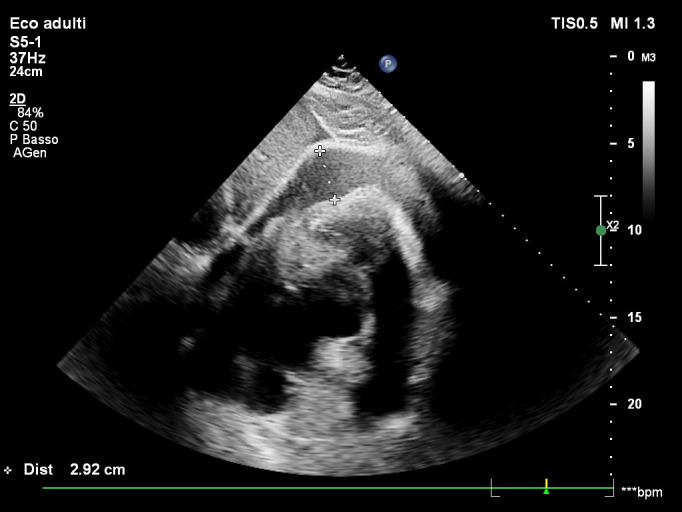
Transthoracic echocardiography (subcostal view) showing a large circumferential pericardial effusion. Subcostal TTE image demonstrating a prominent anechoic pericardial fluid layer encircling the heart; caliper measurement indicates an effusion thickness of approximately 2.92 cm. This view is commonly used at the bedside to rapidly quantify effusion size and support urgent evaluation for tamponade physiology in unstable patients.
